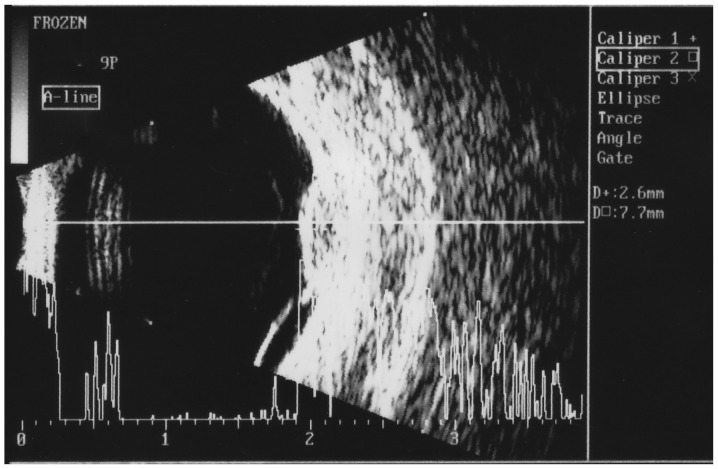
B-scan ultrasound showing a choroidal mass lesion with overlying exudative retinal detachment.
